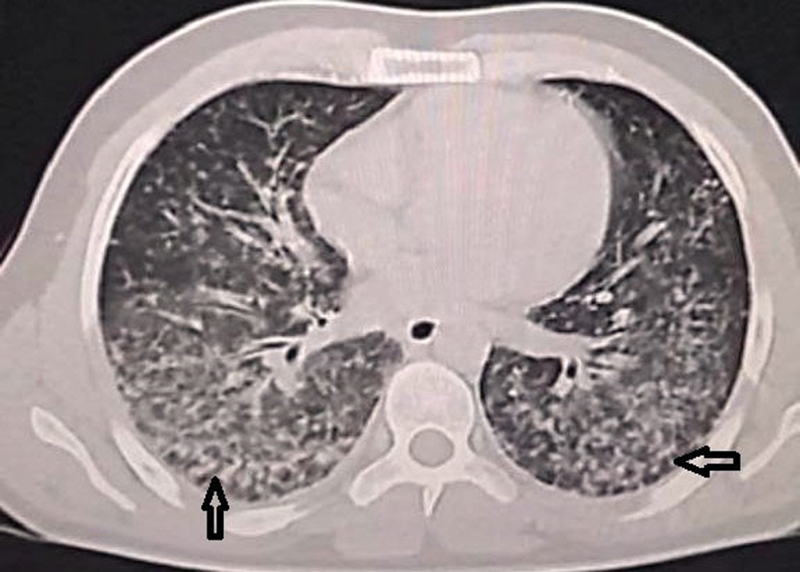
Thoracic computed tomography (CT) showed diffuse infiltration with bronchogram. Arrows point to the miliary nodular pattern and tree-in-bud sign on thoracic CT.
